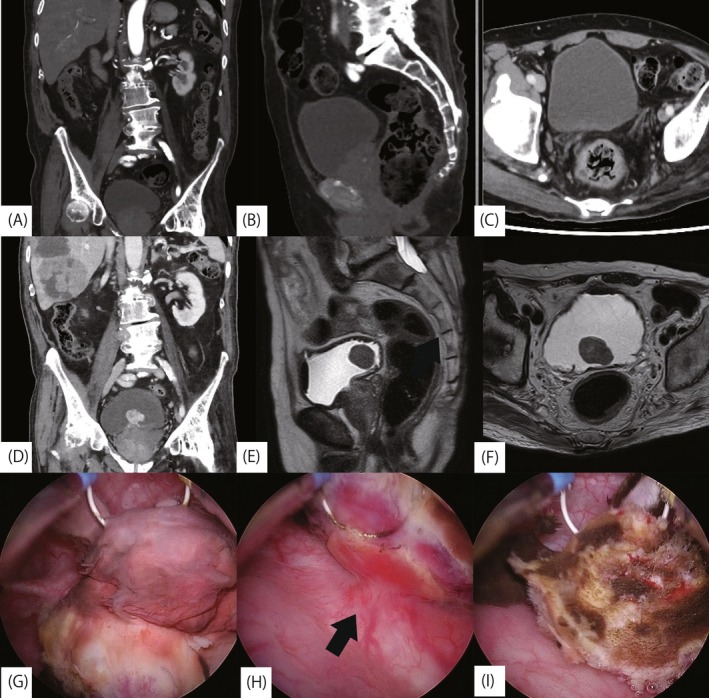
CT images obtained 3 months earlier showed no evidence of a bladder tumor (A–C). At diagnosis, CT revealed a 23 mm tumor on the posterior wall of the bladder (D), and MRI suggested no evidence of obvious muscle invasion (E, F). Intraoperative findings showed a nonpapillary tumor located on the posterior wall of the bladder (G), characterized by a slender pedunculated base (H; indicated by arrow) and a necrotic center (I).
